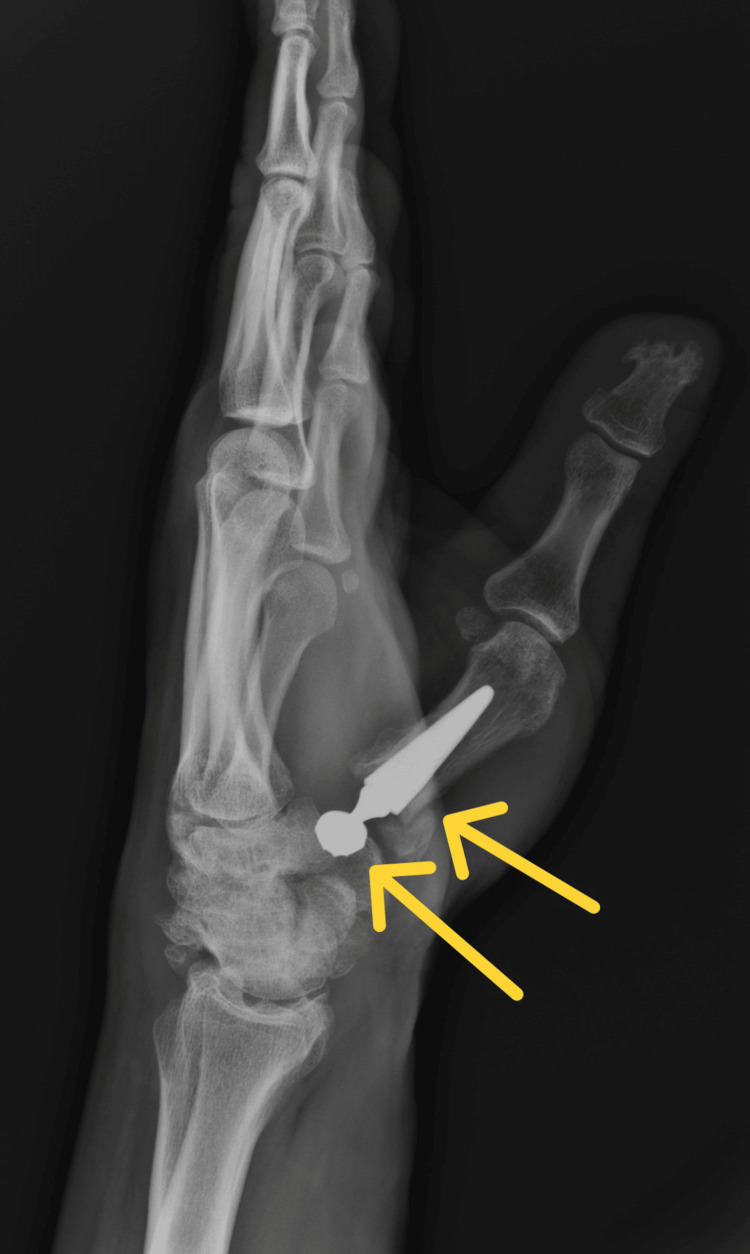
X-ray in lateral projection obtained two years after surgery
